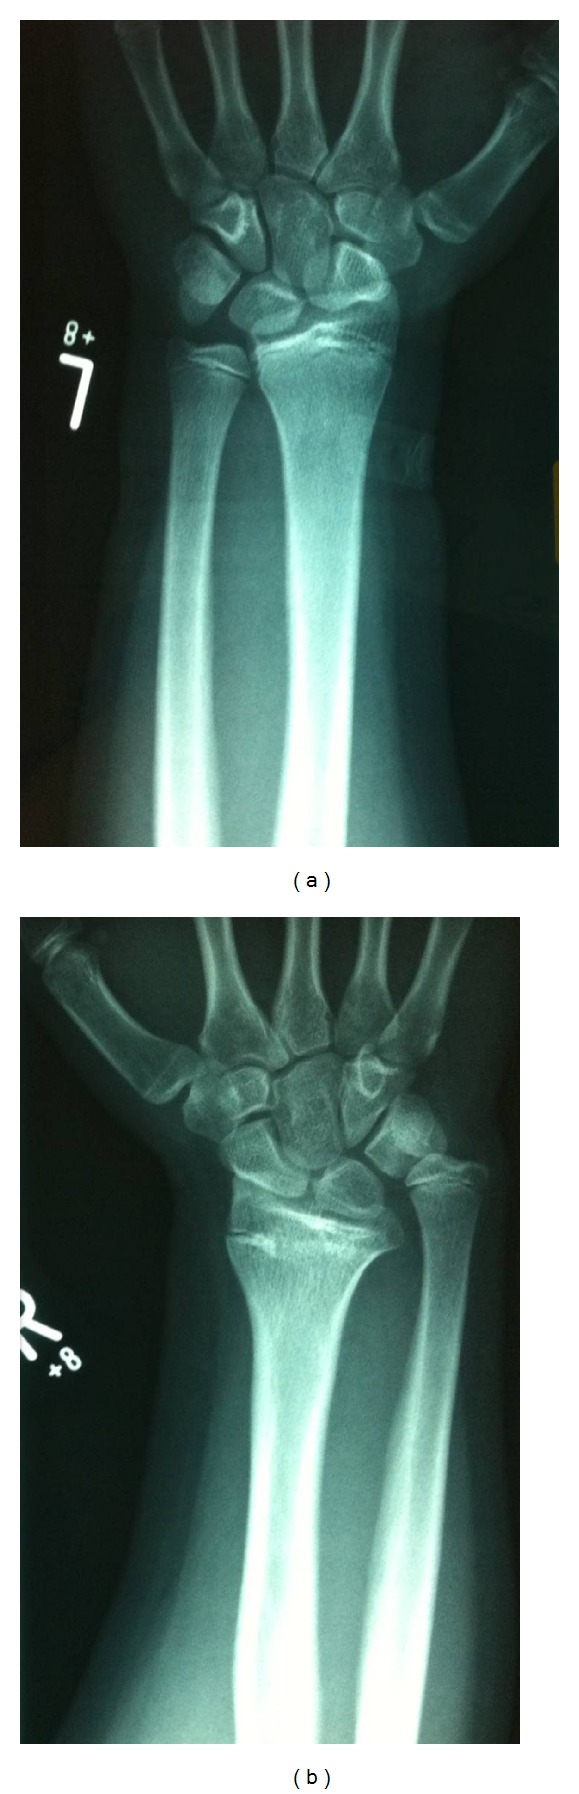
Bilateral anteroposterior radiographs of the distal radius demonstrating distal radius growth arrest on the right side compared to the normal left side.
